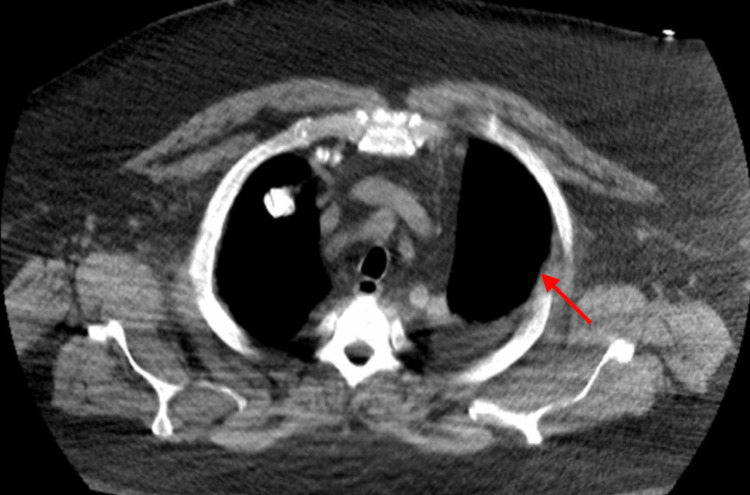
Axial chest CT showing marked interval improvement after extended intrapleural tPA-DNase therapy Follow-up axial computed tomography (CT) of the chest demonstrates marked interval resolution of the previously identified loculated left pleural collection (red arrow), with re-expansion of the left lung after completion of 12 doses of intrapleural tissue plasminogen activator (tPA)-dornase alfa (DNase).
